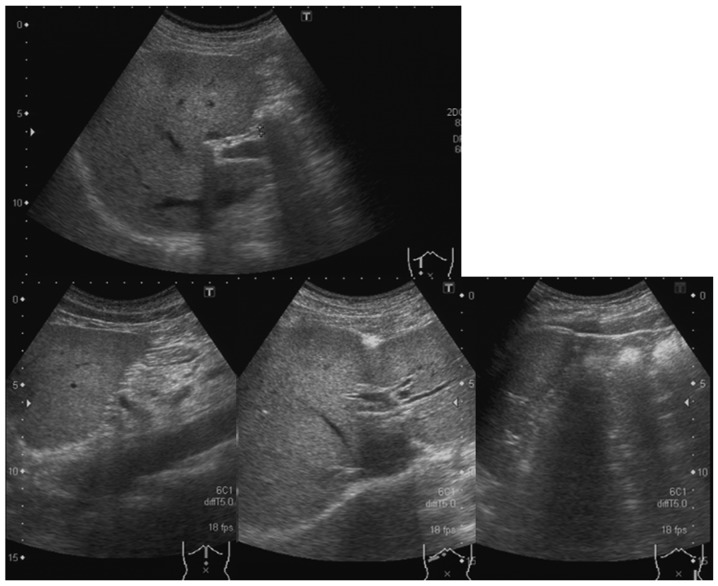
B-mode abdominal ultrasound (US) image on hospital day 2 showing fatty liver-like parenchyma with (A) patchy bright areas. (A,B) No hepatomegaly, (D) splenomegaly, (A–C) mass lesion, (A,C) ascites or distention of the intrahepatic or common bile ducts were observed.
